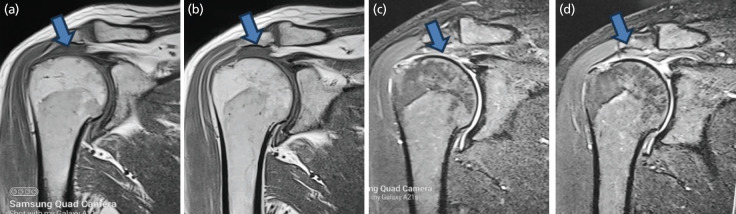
MRI images of supraspinatus tendon (SST) and biceps tendon (BT) thickness in diabetics. MRI Rt Shoulder in diabetic patients, (a and b) arrowhead showing complete tear of Supraspinatus in T1 weighted image, (c and d) arrowhead showing complete tear of Supraspinatus in T2 weighted image.
